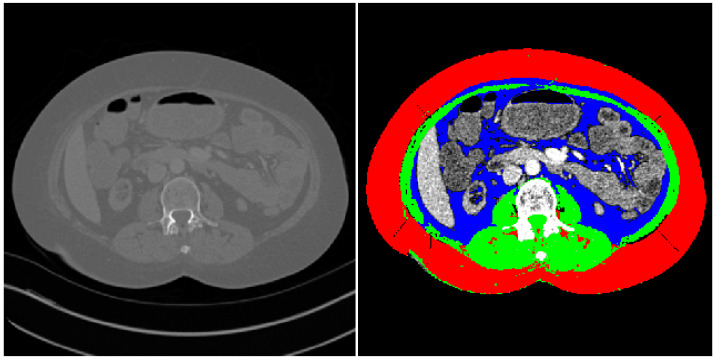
A CT slice at the level of L3 used for assessment of sarcopenia. Points around the peritoneum were traced to enable segmentation of the imported CT slice (left) into the final image with a separating of skeletal muscle and visceral, subcutaneous fat (right). Red = subcutaneous fat, blue = visceral fat, and green = skeletal muscle.
